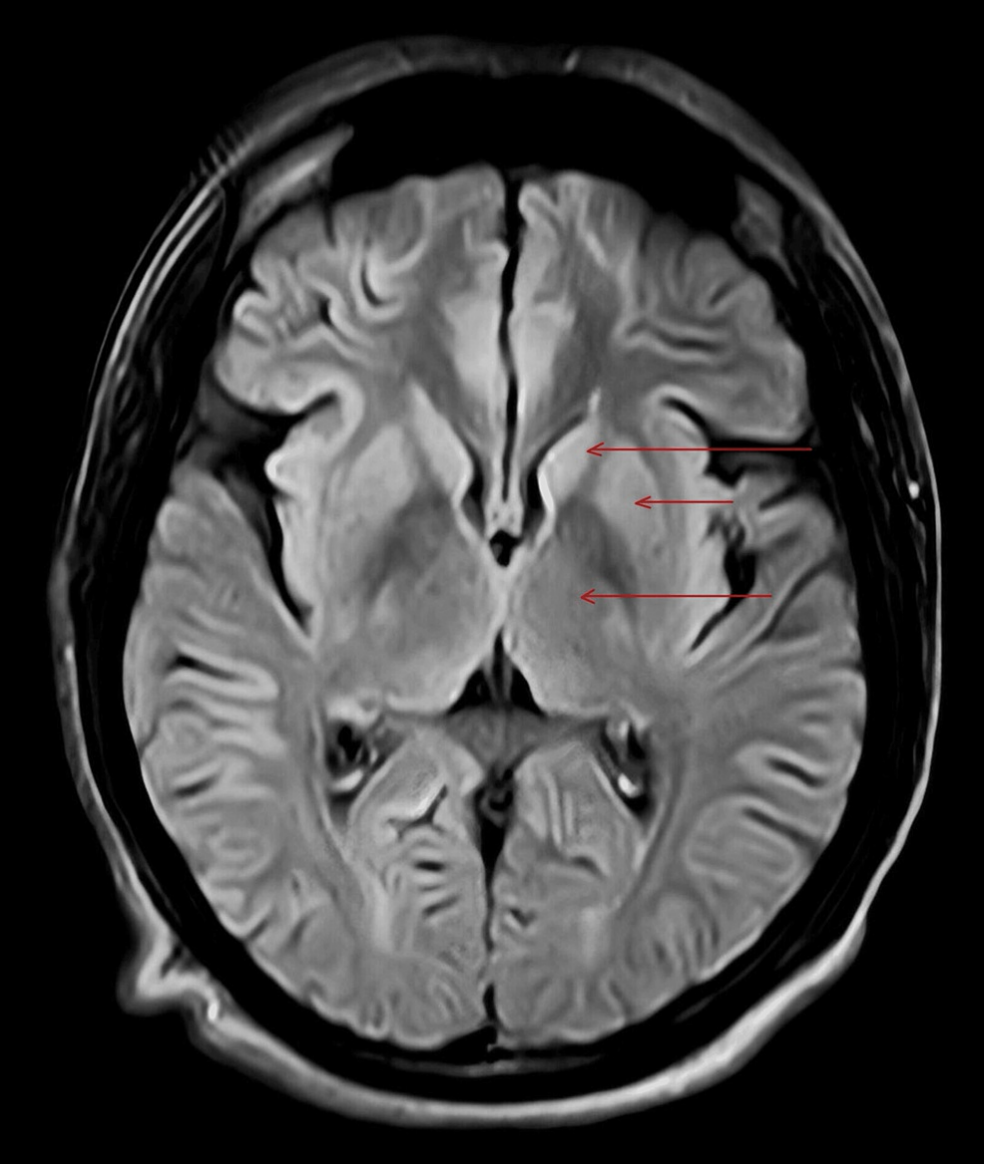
This shows an MRI FLAIR image with no obvious abnormality in the basal ganglia (red arrows showing basal ganglia)
